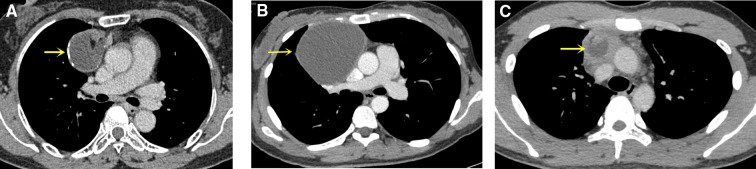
Mediastinal mature teratomas on the CT image. (A) Separation is defined as a well-defined lesion, at a certain distance from the surrounding blood vessels (SVC and IV); (B) Proximation is defined as a clear boundary between the lesion and surrounding organs, and the blood vessel is partially deformed by the mass; (C) Invasion is defined as the non-fatty lesion invaded the surrounding vessels. The yellow arrow points to MMT. CT, computerized tomography; SVC, superior vena cava; IV, innominate vein; MMT, mediastinal mature teratoma.
